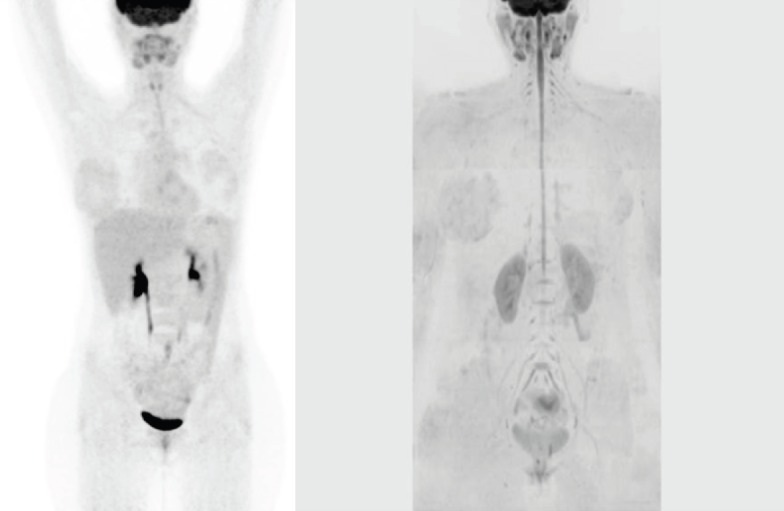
(a) Coronal MIP FDG-PET/CT performed six months after treatment demonstrating the absence of any residual abnormal activity. (b) Post-treatment coronal, whole-body grayscale inverted MIP DW-MRI showing no nodal or extranodalresidual disease.
